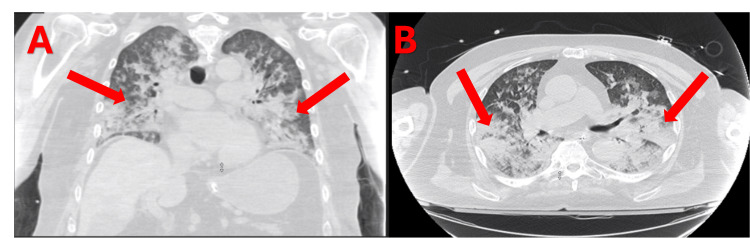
CT chest depicting patchy opacities bilaterally in coronal (A) and transverse (B) views.
